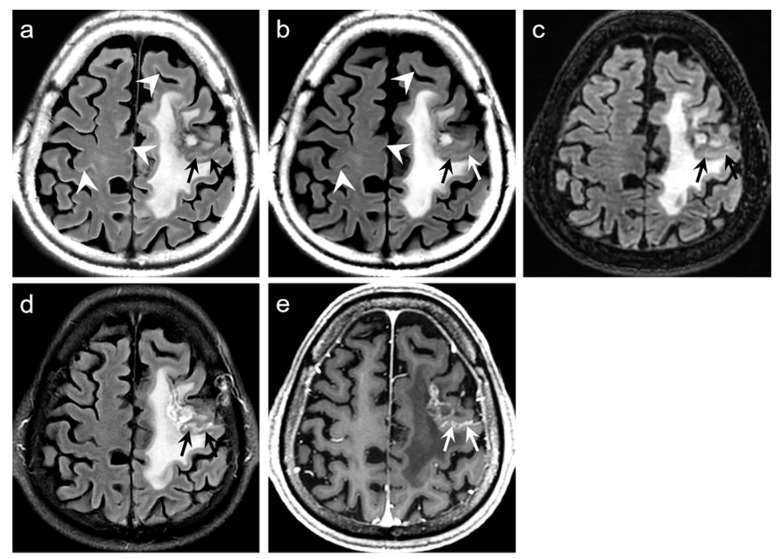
Representative case of preservation of true sulcal lesion in patient with brain and leptomeningeal metastases from lung cancer. Leptomeningeal metastases showing sulcal hyperintensity (arrows) on the native synthetic FLAIR (a) are preserved on DL-FLAIR (b), whereas surface hyperintense artifacts on the native synthetic FLAIR (arrowheads on a) are removed on DL-FLAIR image (b). The leptomeningeal metastases are conspicuously delineated on 3D-FLAIR (c), and the corresponding lesions show prominent enhancement on Gd-enhanced FLAIR (d) and Gd-enhanced 3D T1-weighted image (e).
